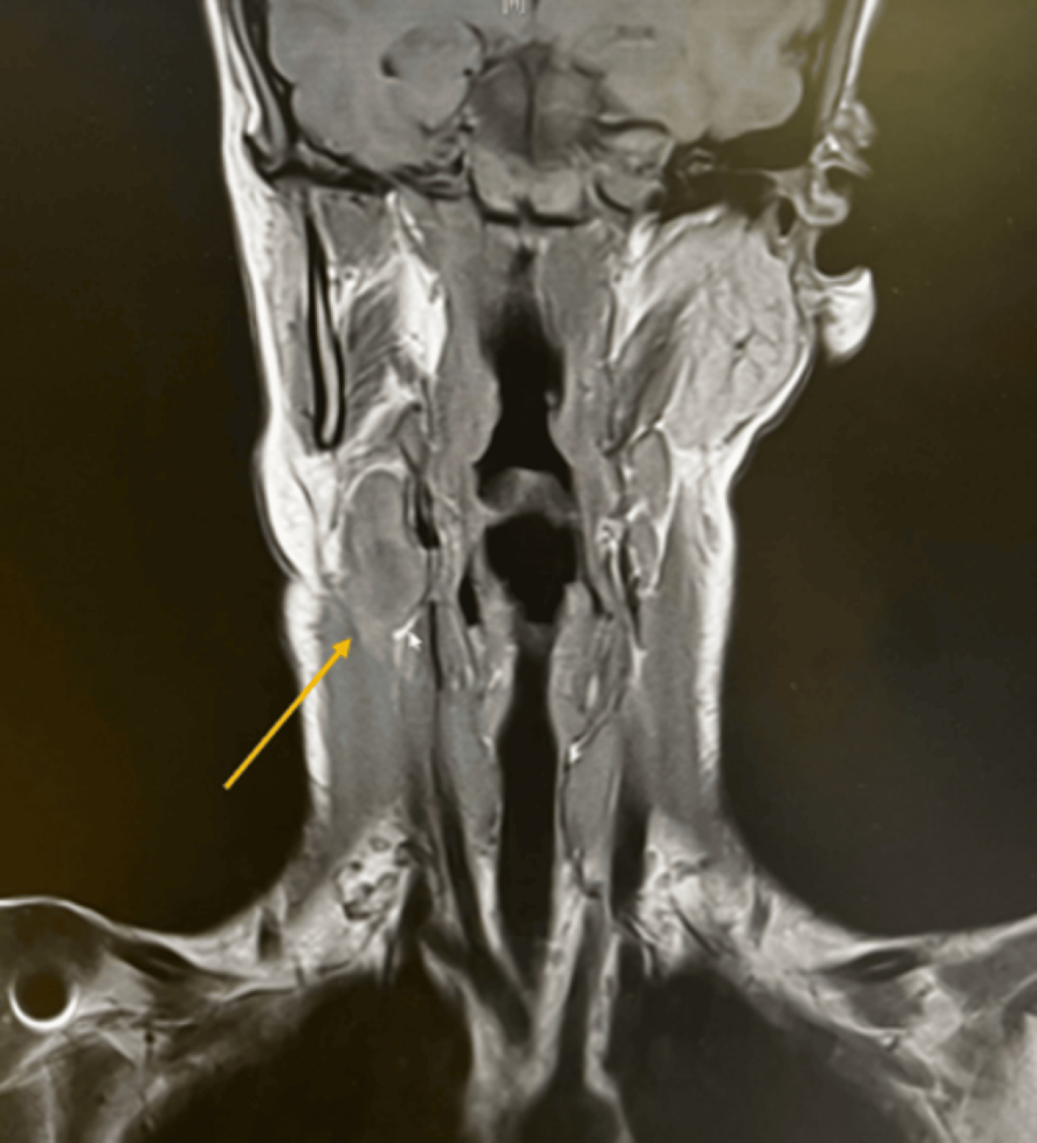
Follow-up MRI one day after ultrasound-guided drainage showing no recollection of fluid.
